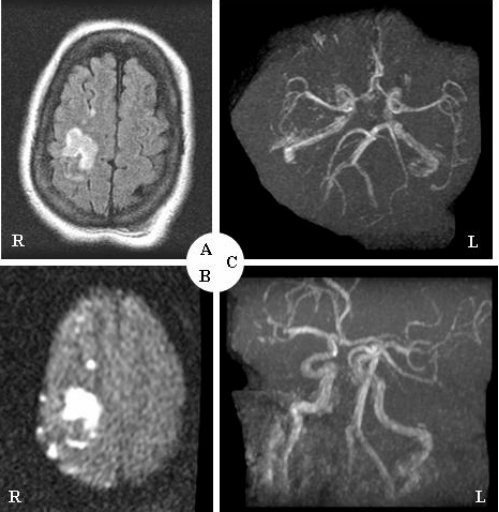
Axial T_1_-weighted MRI shows acute infarcts in the right caudate body, and the frontal and parietal lobes. (A), The diffusion weighted image (DWI) presents an abnormal signal corresponding to the restricted diffusion in the right hemisphere. (B), MRA shows no aneurysm, stenosis, or abnormal flow in the visualized vessels of the Circle of Willis, the carotid arteries, and the vertebral arteries. (C).
